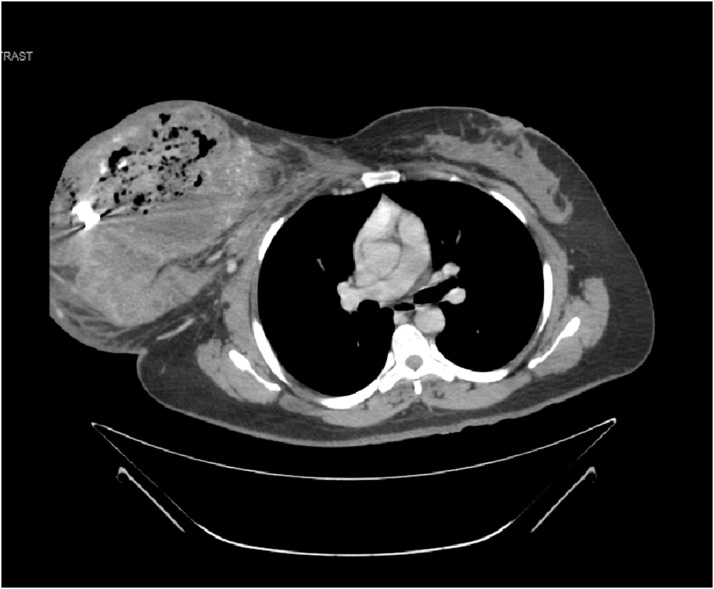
CT-Scan chest Transvers section showing huge heterogeneous right breast mass.
